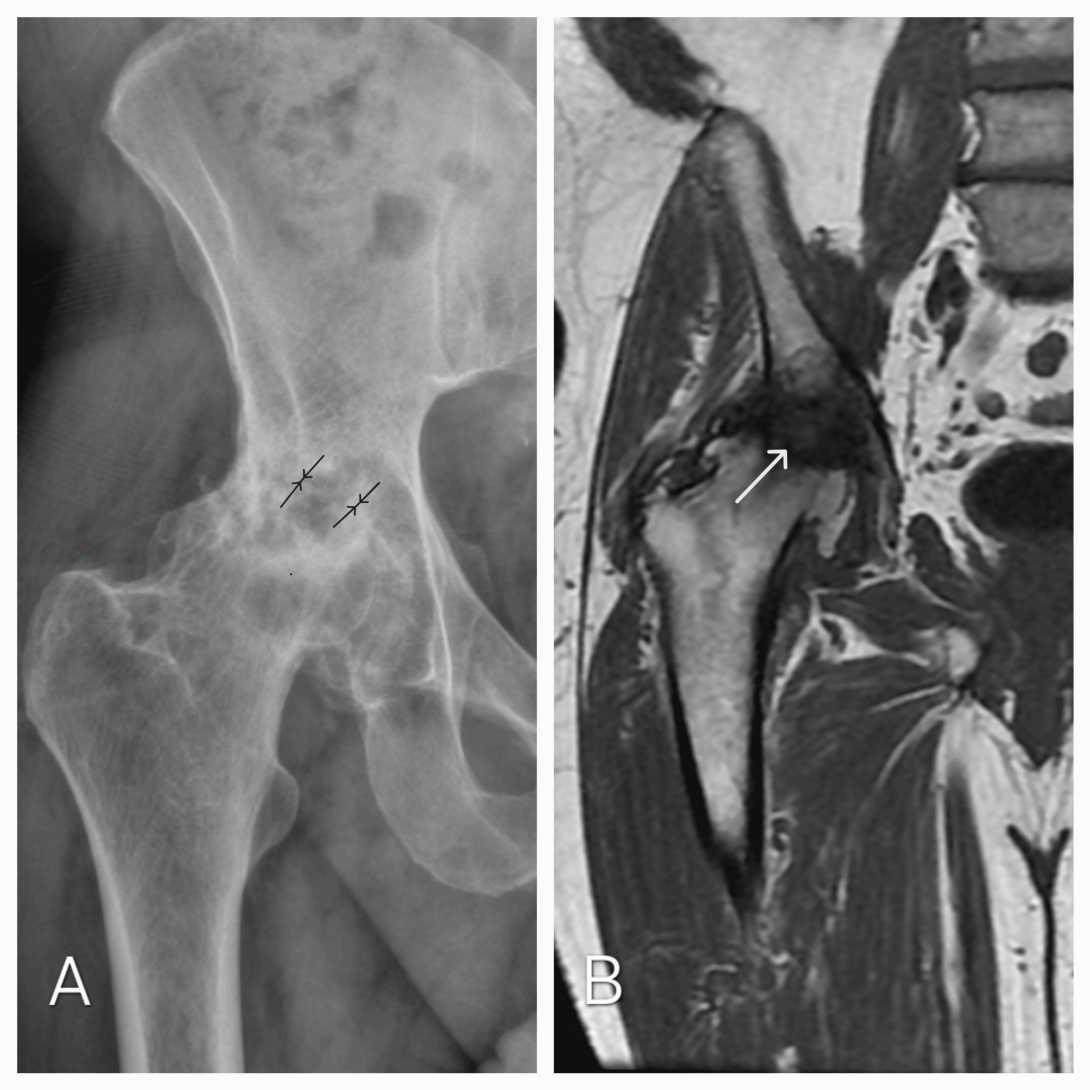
MRI and X-ray showing osteonecrosis of the femoral head (stage 4) (A) X-ray: severe coxofemoral joint narrowing (arrows) with subchondral cysts and femoral head flattening. (B) MRI: coronal view showing the hypointense necrotic area (arrow), deformity of the right femoral head, loss of sphericity, and coxofemoral joint narrowing.
